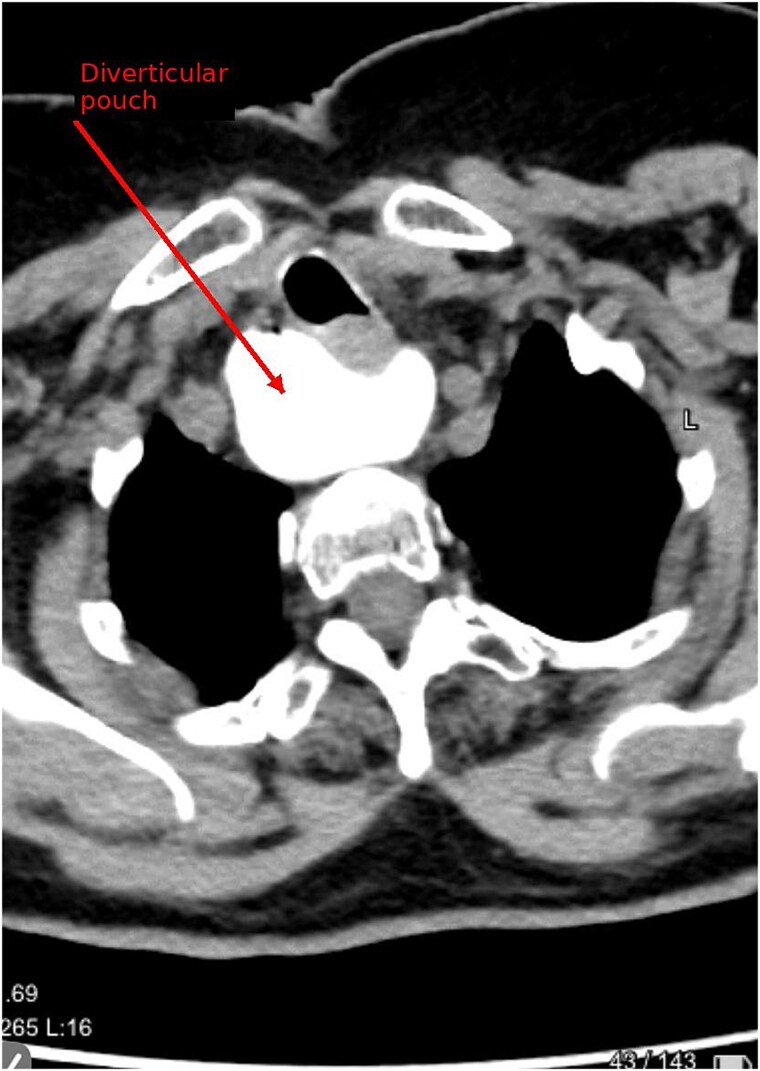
Contrast-enhanced CT (axial) demonstrating the diverticular pouch at the thoracic inlet level.
